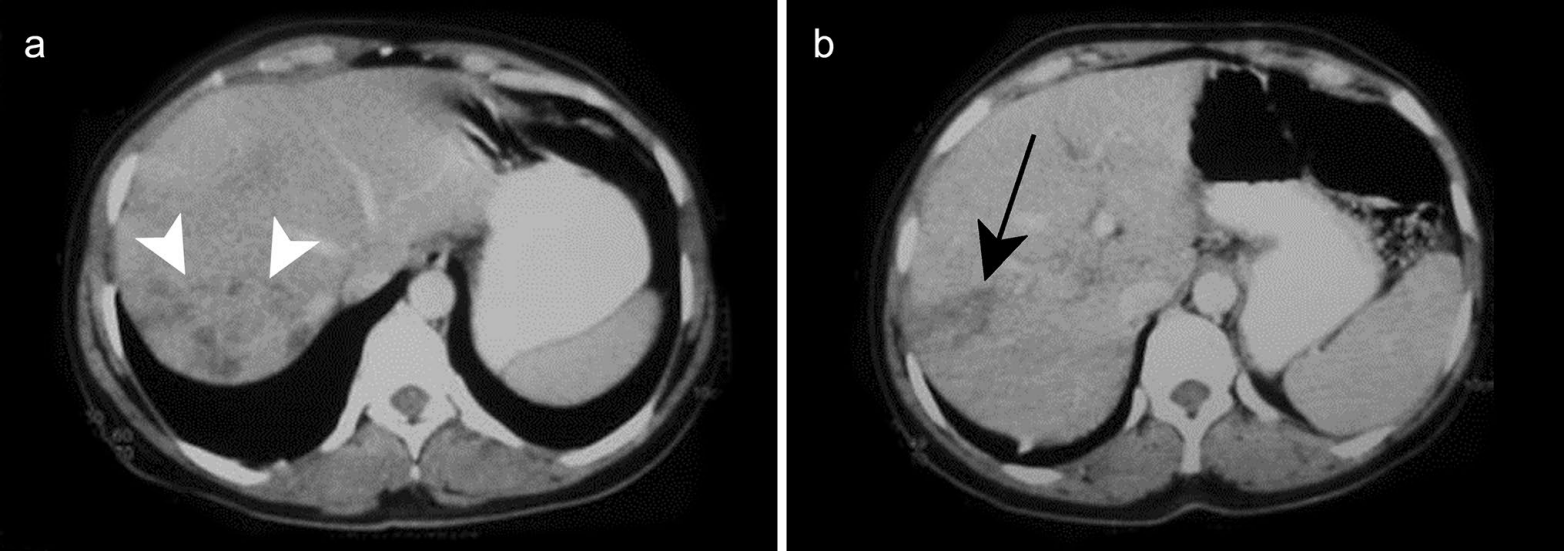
Fascioliasis in a 42-year-old female patient with right upper abdominal pain and low-grade fever. Axial contrast-enhanced CT (a, b) show patchy ill-defined hypoattenuating lesions with subcapsular (white arrowheads) and periportal distribution (black arrow)
